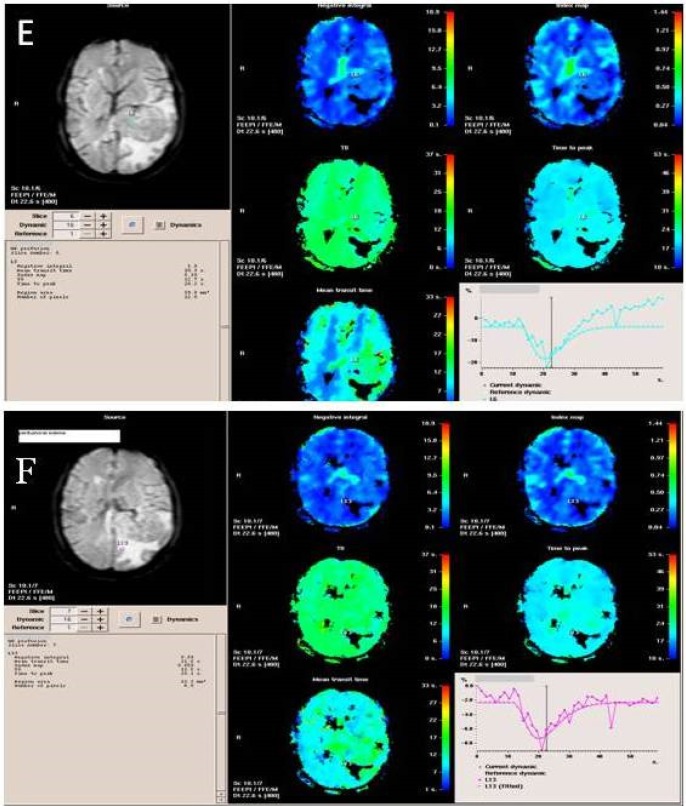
A 40-year-old male with left intraventricular meningioma (benign) A. Axial T1W image showing well defined hypointense lesion in atrium of left lateral ventricle B. T2W image showing hyperintense lesion with peripheral edema C. On contrast enhanced axial T1W images lesion showing homogenous intense enhancement. D. Axial DWI and corrosponding ADC map showing diffusion restriction with minimum ADC value of being 0.733×10^−3^ mm^2^/_s_ E. T2W* first pass perfusion images showing colour coded rCBV maps along with time signal intensity curve and various parameters with rCBV(negative integral) being 1.9 mL/100 g within the tumor F. T2W* first pass perfusion images showing colour-coded rCBV maps along with time signal intensity curve and various parameters with rCBV being 0.69 mL/100 g in peritumoral edema
